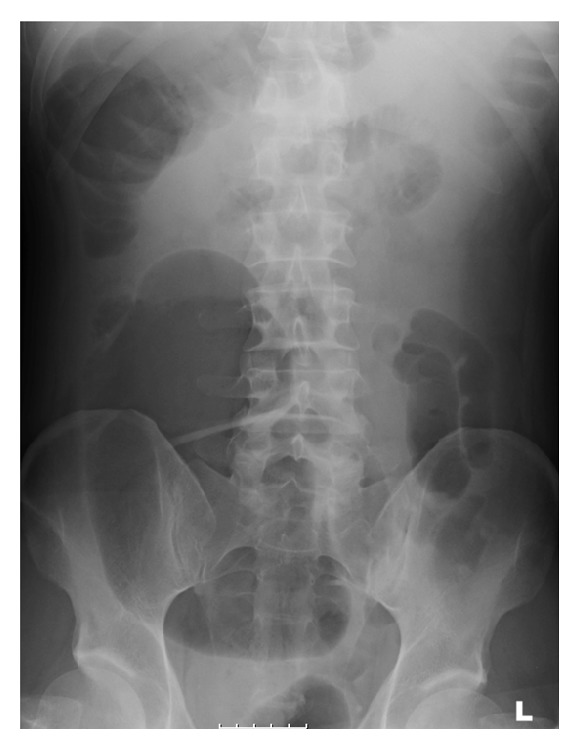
Abdominal X-ray at presentation showing hugely dilated caecum and transverse colon with paucity of gas in the descending colon.
